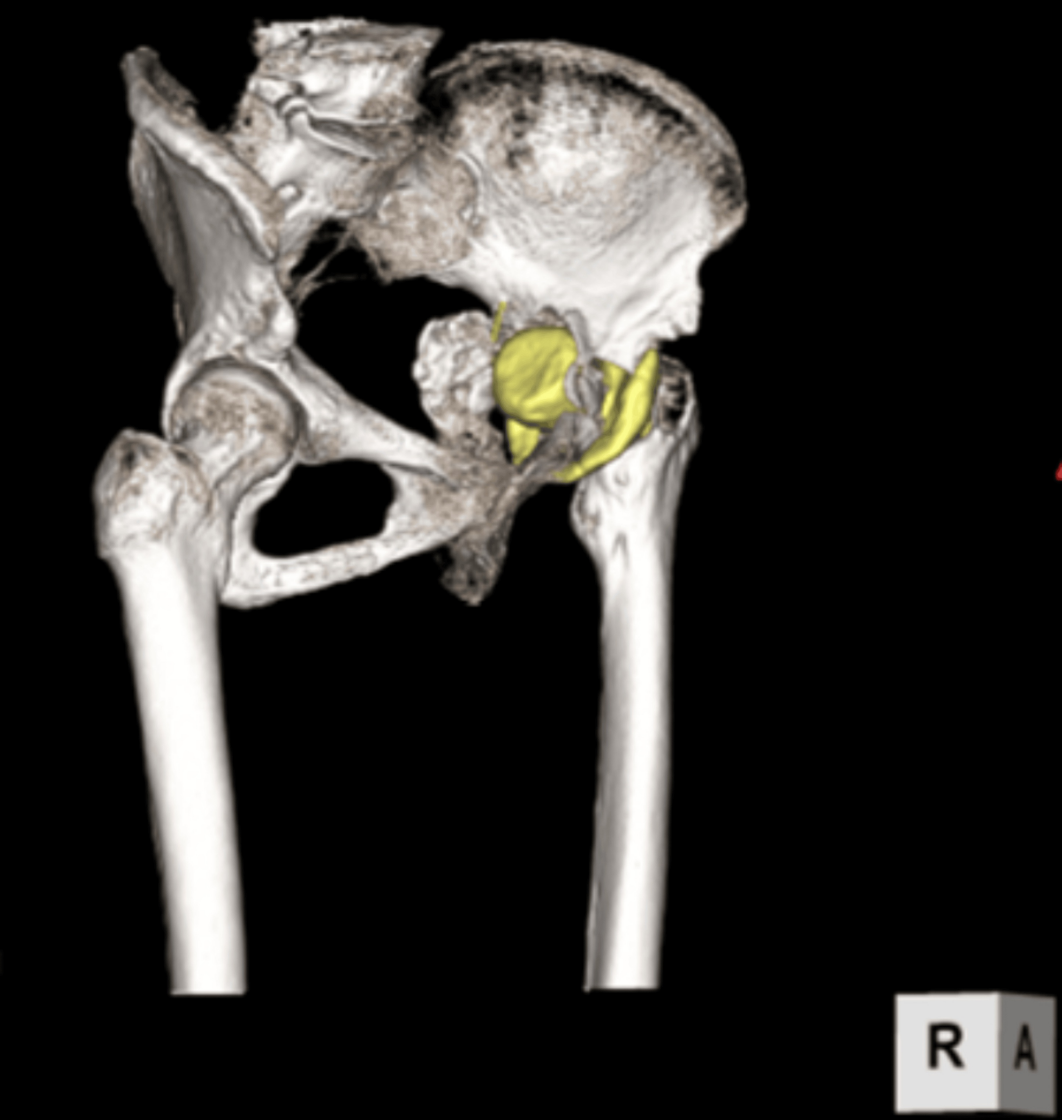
CT image of the hip after the first-stage removal The yellow region represents the cement spacer. Pelvic discontinuity with a large bone defect was observed.
